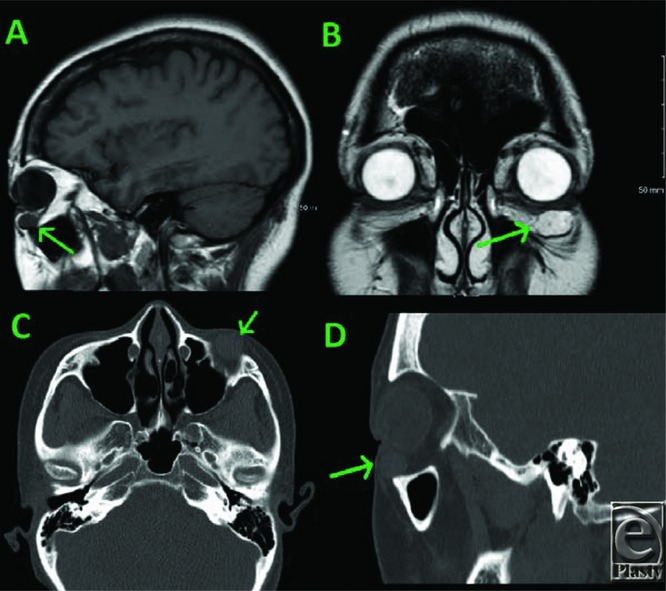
(a, b) T2- and T1-weighted magnetic resonance images. (c, d) Computed tomographic w/o contrast images of the orbital mass. Green arrow points to the orbital mass.
